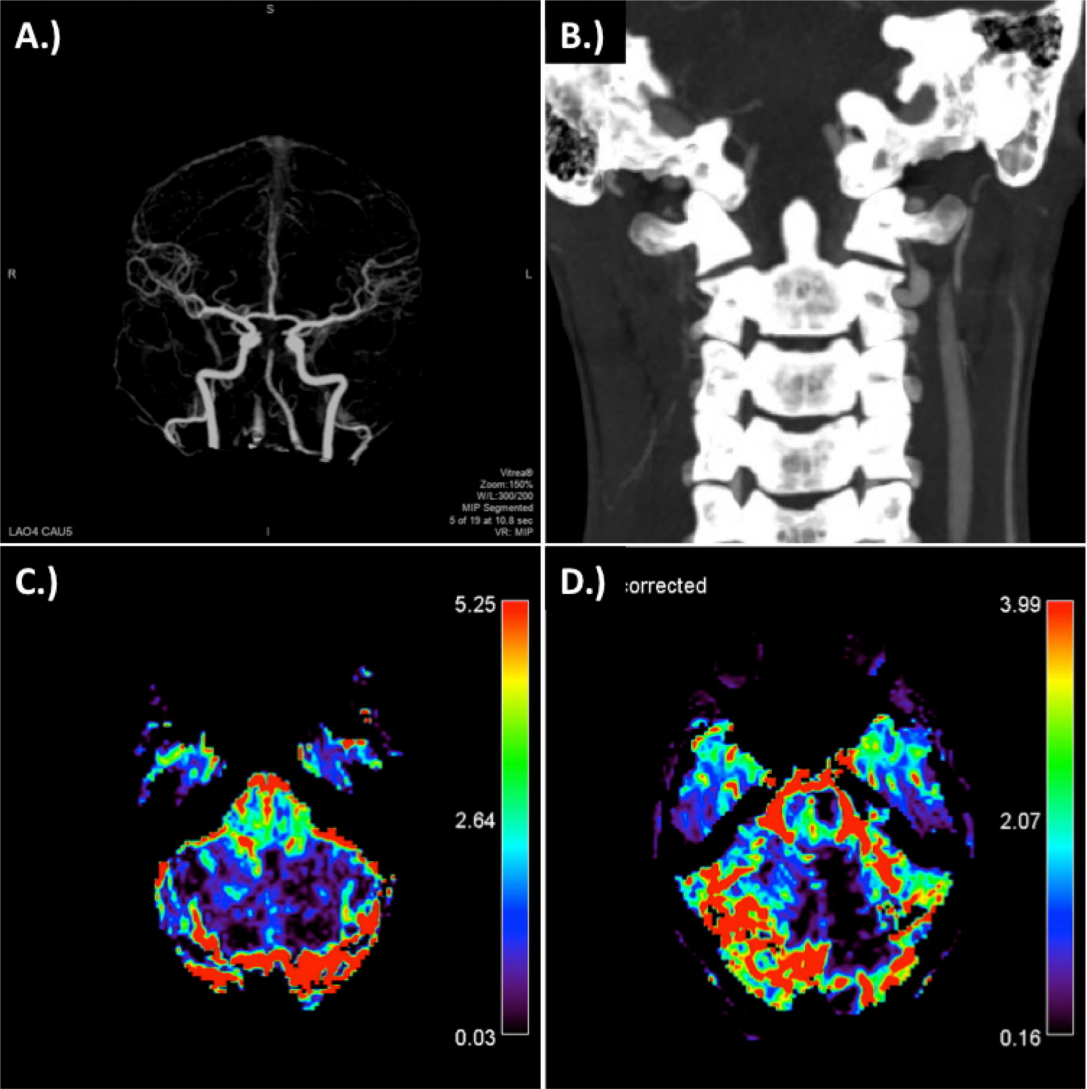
CT Stroke Protocol at time of presentation. A.) 4D CTA demonstrating top of the basilar occusion and poor flow in the right vertebral artery; B.) coronal CA demonstrating dissection of verterbral arteries at the C1–C2 level, particularly prominent on the right; C.) relative blood volume; and D.) corrected relative blood volume.
